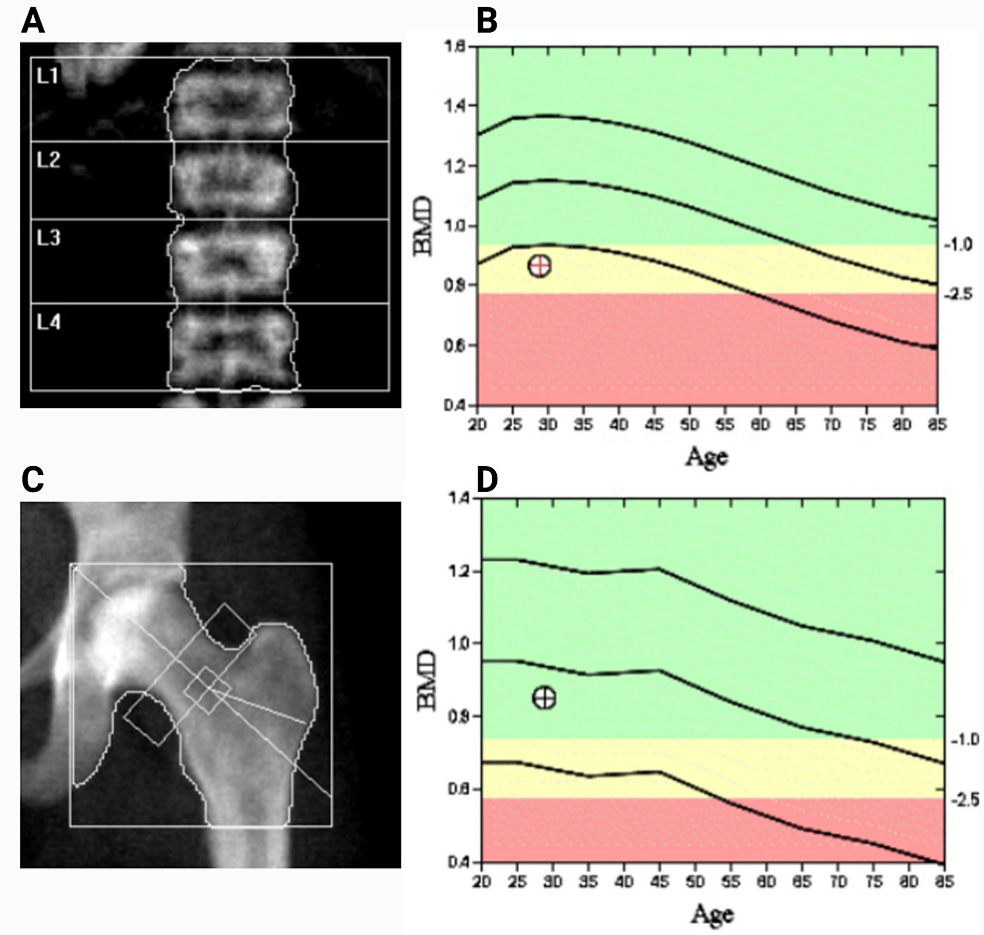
Dual-energy X-ray absorptiometry (DEXA) scan of the lumbar spine (A) with corresponding densitometry (B) and the hip and femoral neck (C) with corresponding densitometry (D) regions showing low bone mineral density as indicated by the mark on densitometry (B) lying in the yellow range. BMD: Bone mineral density
